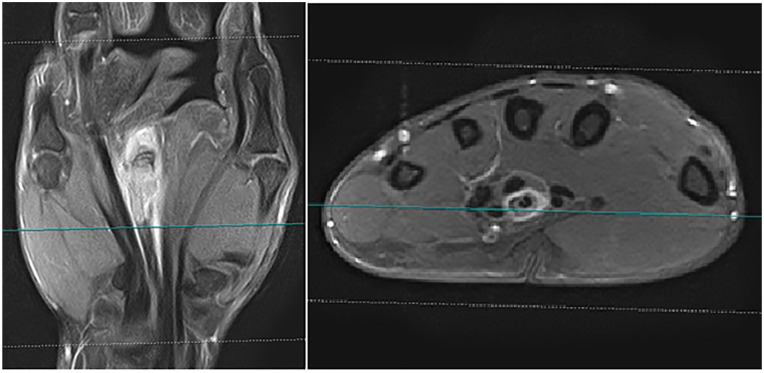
Magnetic resonance imaging with the contrast of the hand. Epithelioid sarcoma spreading along the flexor tendon of the third finger.
